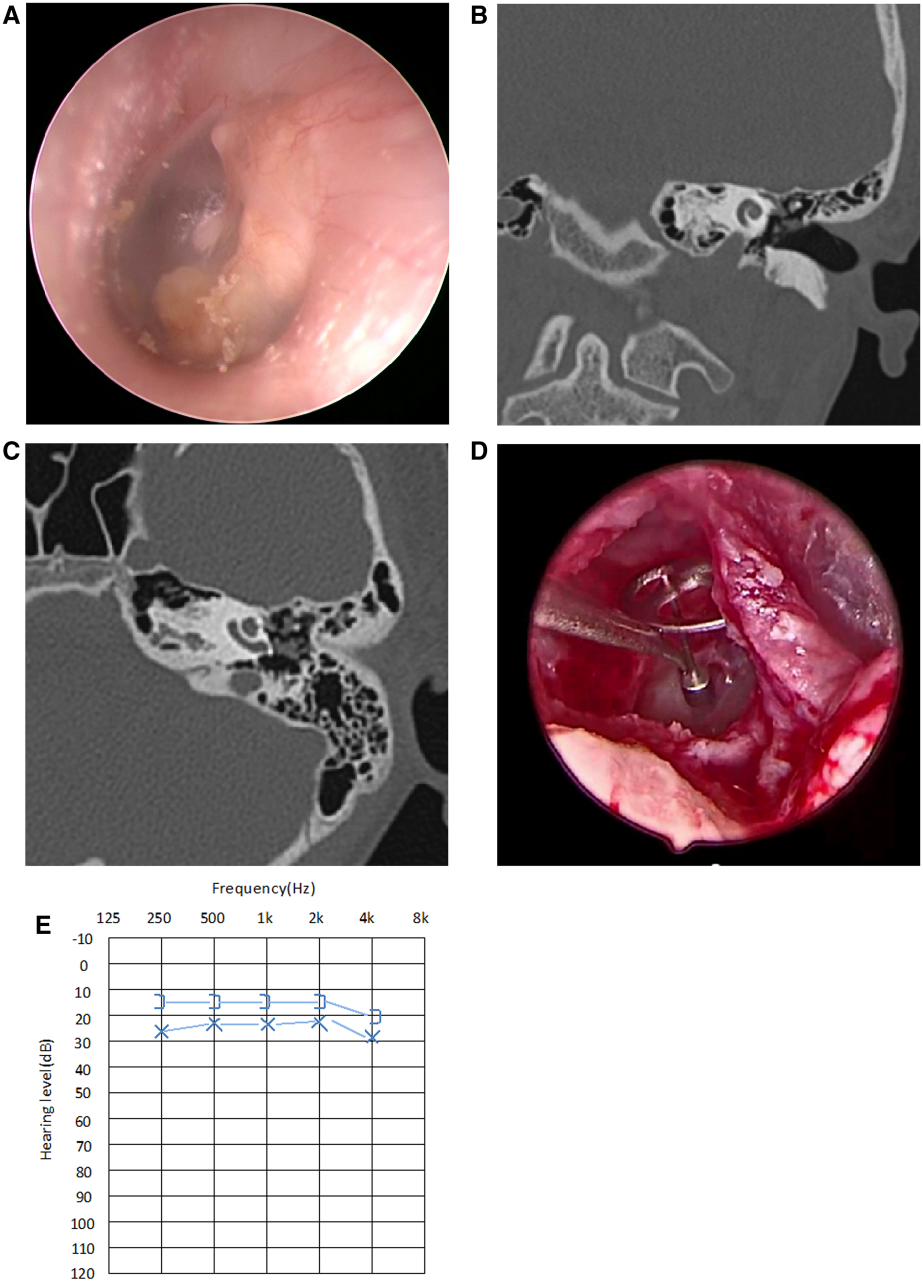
Typical case of stage III CMEC. (A) Endoscopic findings revealed a white mass involving multiple quadrants of the tympanic membrane; (B,C) CT showed a soft tissue density in the left side of the middle ear, with the ossicular chain impaired, but without involving the mastoid; (D) After complete removal of the congenital cholesteatoma, the stapes superstructure was absent, so TOPR was conducted for hearing reconstruction; (E) Postoperative audiogram at the 3-month follow-up showed a mild conductive hearing impairment, with 26.3 dB HL in air conductance, 16.3 dB HL in bone conduction, and 10 dB HL in the air-bone gap.
